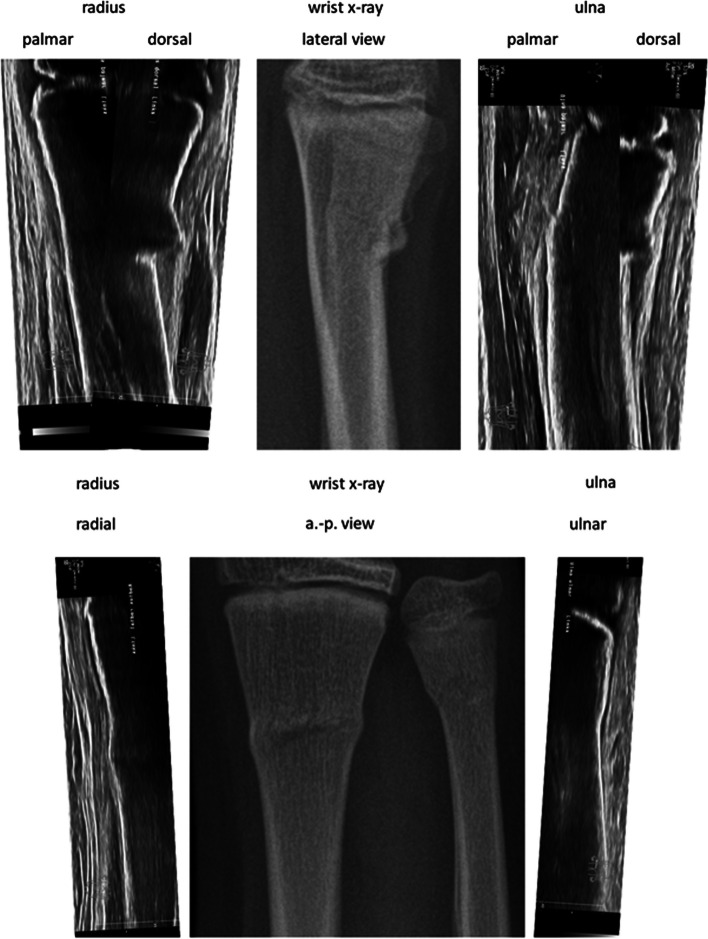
Merged, cut, and sized Wrist-POCUS of distal forearm buckle with corresponding X-rays for comparison
